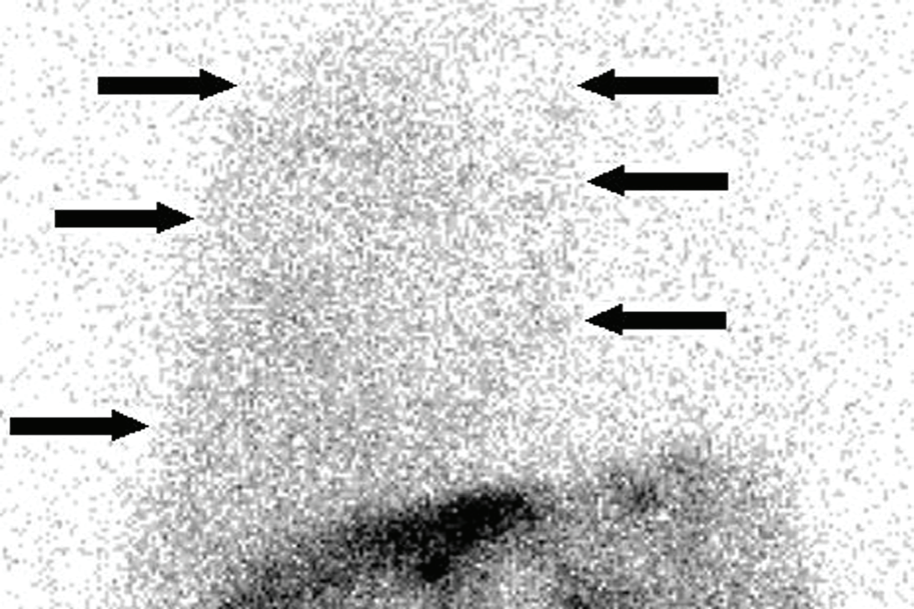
Peritoneal scintigraphy of the case 1 at 3 h: at 10 min, the tracer was diffused in the abdominal region, which confirmed that it was injected properly in the peritoneal cavity; at 3 h, the tracer was seen in the right side of the thoracic region (i.e. pleural space) (arrows). This image suggested peritoneo-pleural communication and hepatic hydrothorax.
